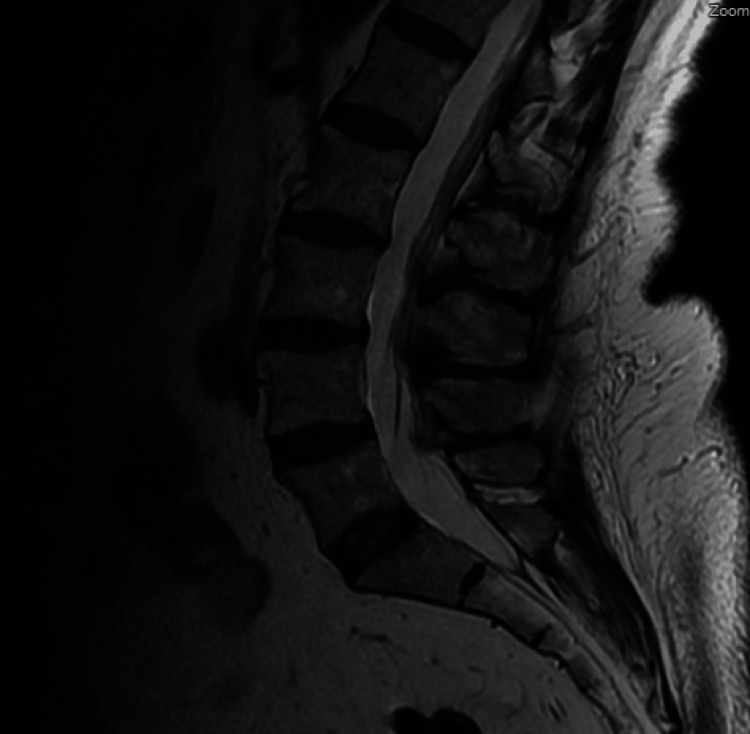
MRI lumbar spine showing minimal degenerative changes
